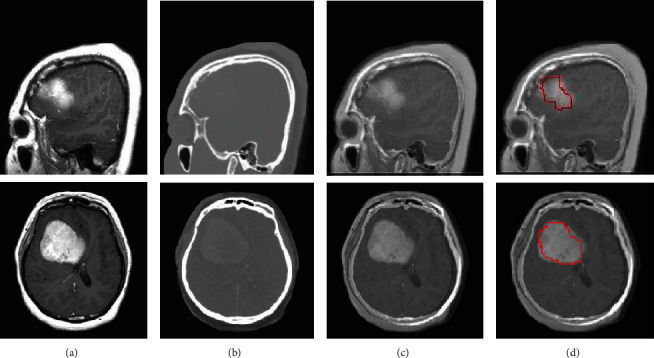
Extraction of brain tumor images. (a) Input-MRI image. (b) Input-CT image. (c) Fused MRI-CT image. (d) Tumor outline.
